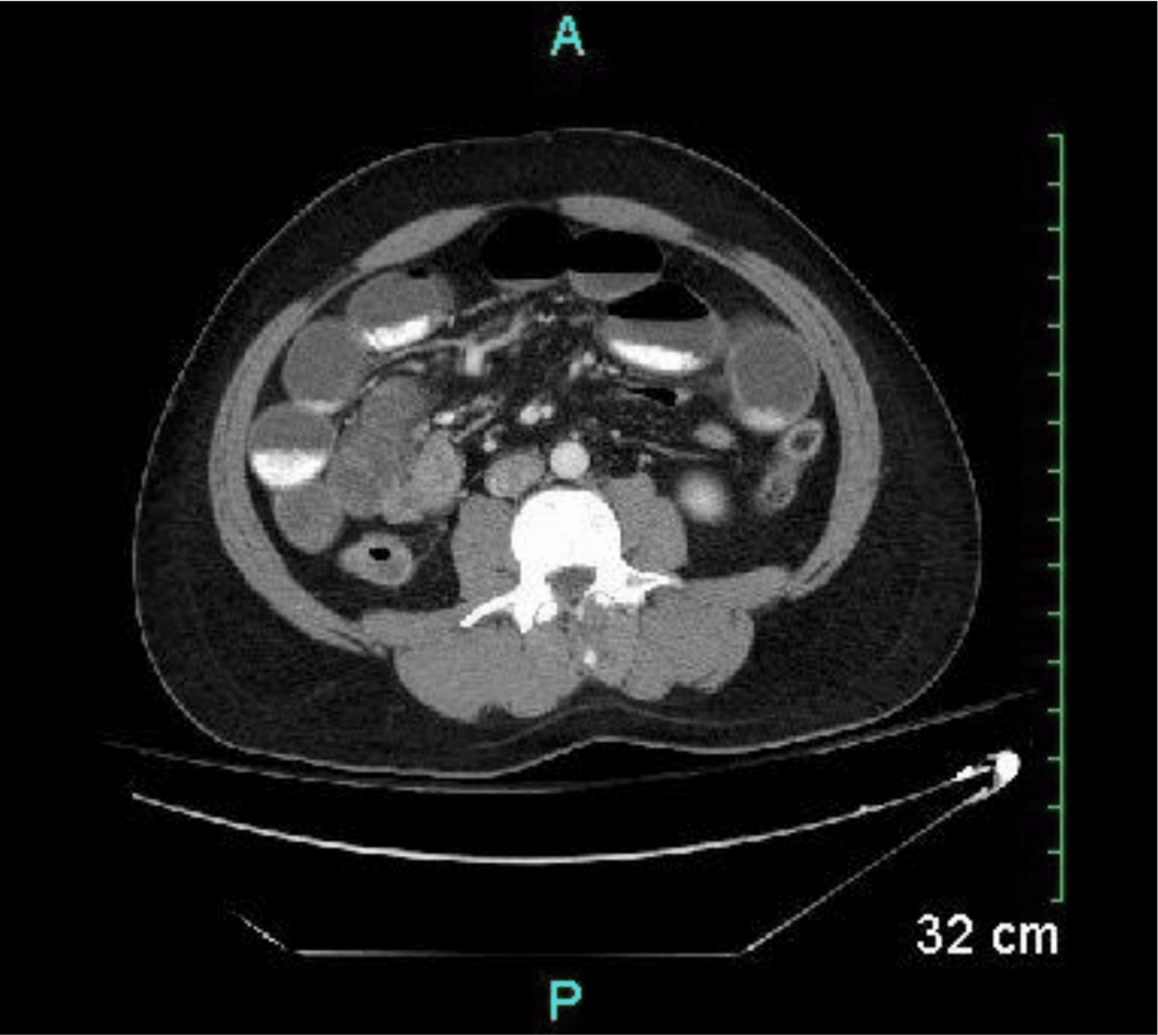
CT Scan of Abdomen and Pelvis with Contrast. CT scan of the abdomen and pelvis with contrast showing a partial small-bowel obstruction characterized by dilated loops of small bowel throughout the abdomen, as well as enteritis characterized by wall thickening involving multiple loops of small bowel.
